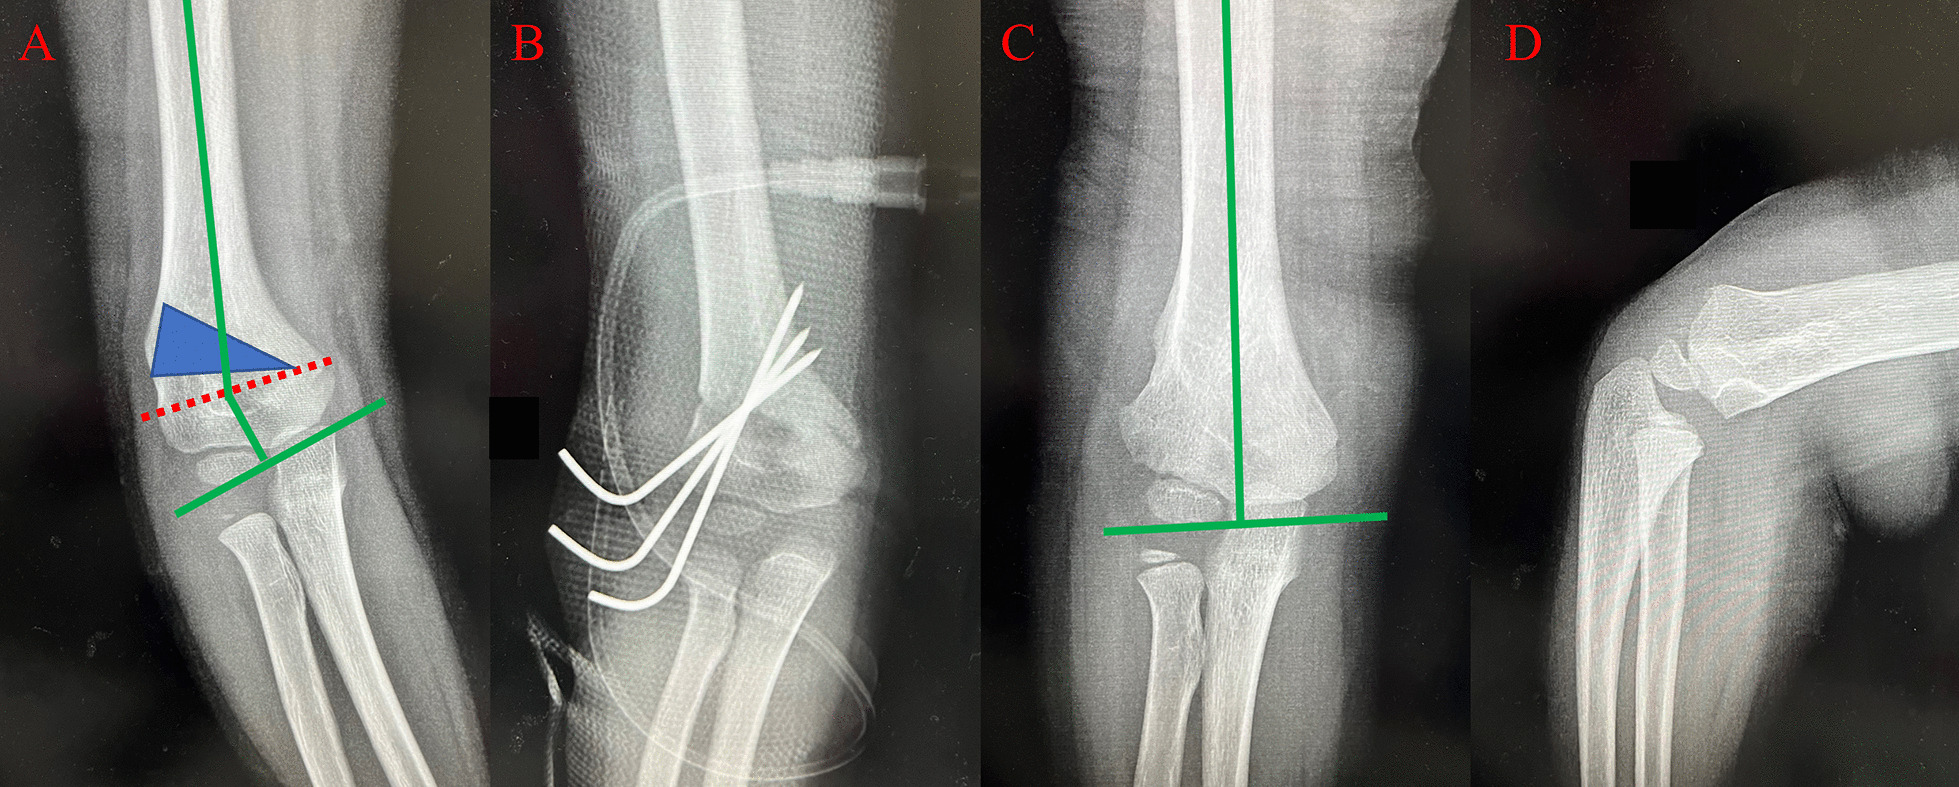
Representative case of a 5-year-old male with right cubitus varus deformity. A Shows the design of the osteotomy. B Shows the antero-posterior radiographs of the osteotomy on the 2nd postoperative day. C, D Show the antero-posterior and lateral sides of the patient at 11 months postoperatively
